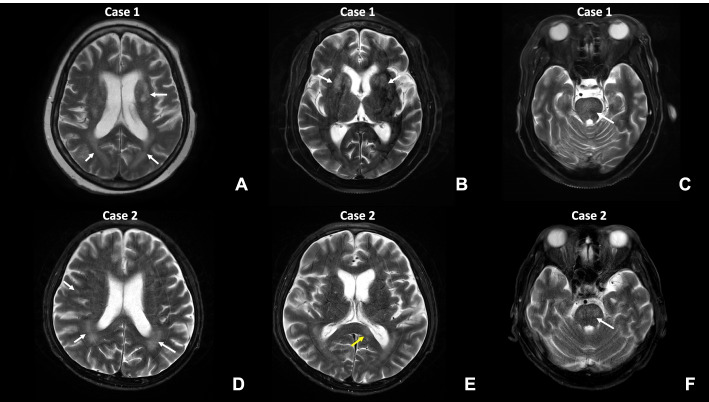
A, B, C T2-weighted brain MRI of case 1 revealed multiple high signal intensity lesions (arrows) in the white matter with diffuse high signal intensity of the optic pathway and central pons (arrow). D, E, F T2-weighted image of case 2 showed high signal intensity lesions (arrows) in the white matter, the splenium of the corpus callosum (yellow arrow) and the pons (arrows)
